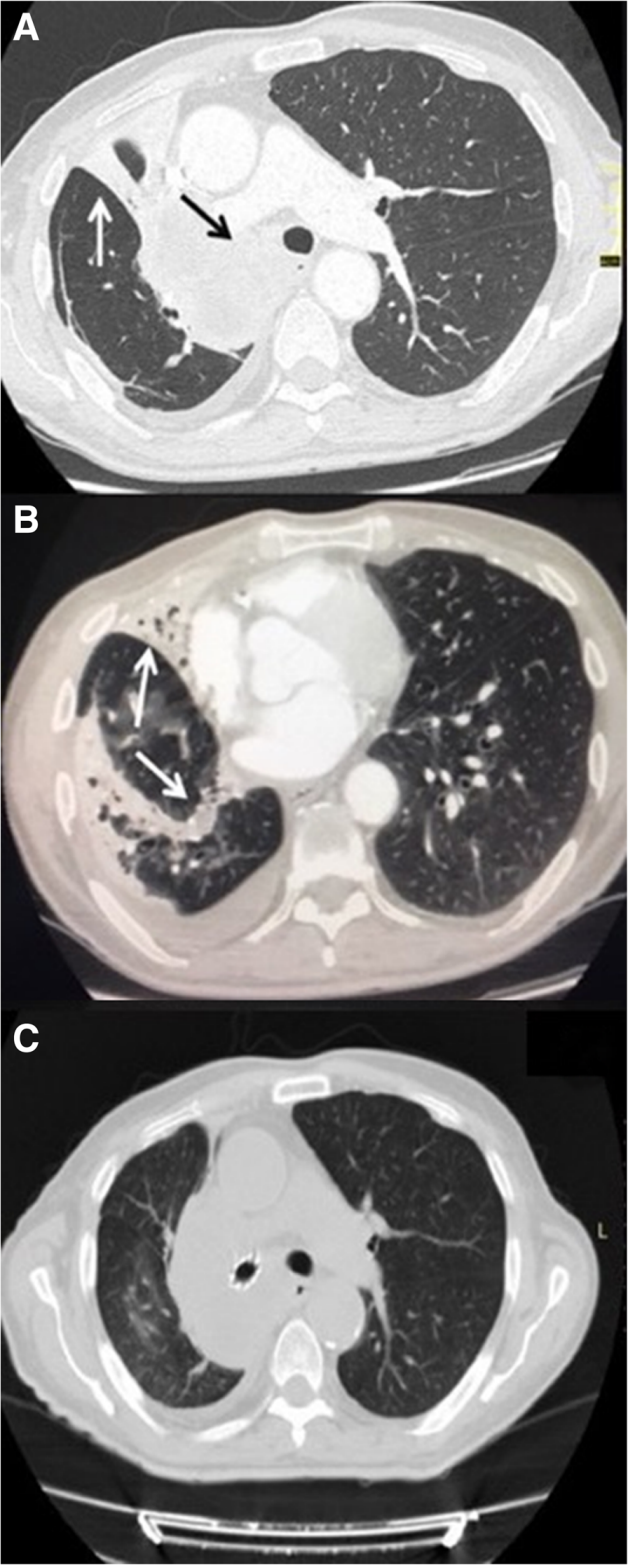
Chest computed tomography scan at the admission, showed the obstruction of the right main bronchus (black arrow) (a), the atelectasis of the middle lobe and the pneumonia of lower lobe (white arrow) with pleural effusion (b). The endoscopic recanalization with stent placement allowed to obtain the resolution of atelectasis and lung infection (c)
